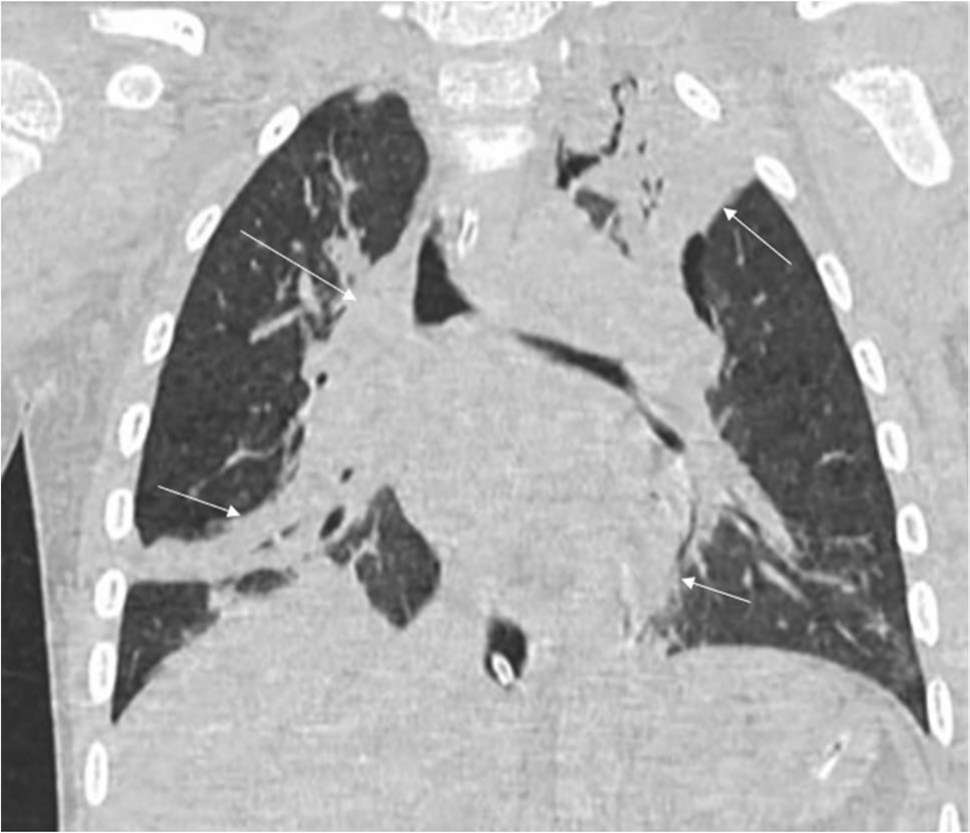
Coronal non-contrast-enhanced lung window computed tomography image in a 3-year-old boy affected by psittacosis who presented with severe dyspnea. The image shows multiple bilateral lung consolidations (*arrows*) (figure from “A pediatric case of *Chlamydia psittaci* caused severe Acute Respiratory Distress Syndrome (ARDS) in Italy” by Marchese et al.; licensed under CC BY; original annotated)
